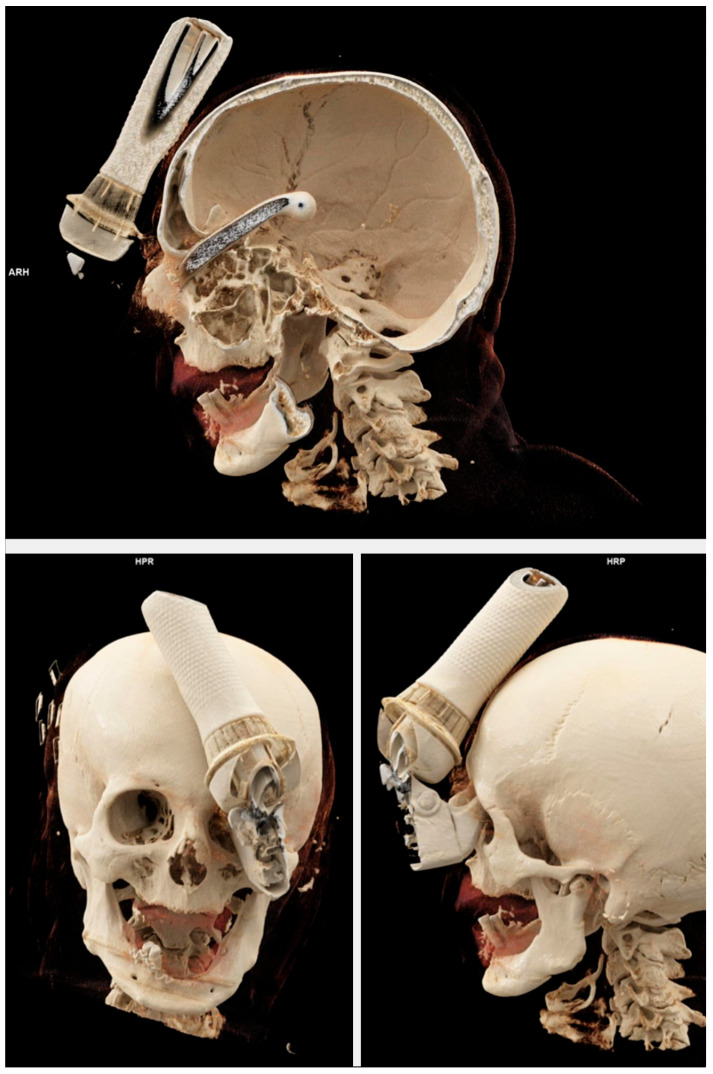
Three-dimensional bony reconstruction from CTA demonstrating a transorbital intracranial injury caused by an e-scooter brake handle.
